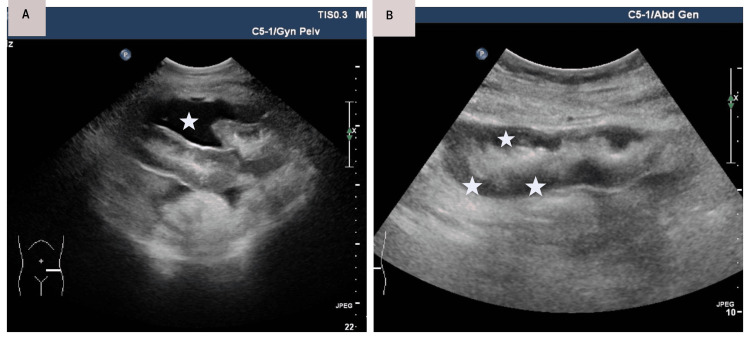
Ultrasound (USG) of the abdomen and pelvis (white asterisk marks) demonstrating ascites (A) and bowel wall thickening (B).
